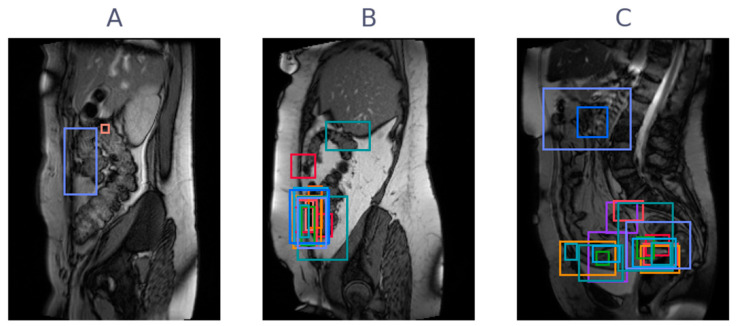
Three examples of cine-MRI slice with varying levels of agreement between observers. Annotations are overlayed as boxes, with a unique color per observer. (A) A slice with high agreement for being negative (only two observers annotated), (B) a slice with high agreement for being positive in the same location, and (C) a slice with many positive annotations but in varying locations.
